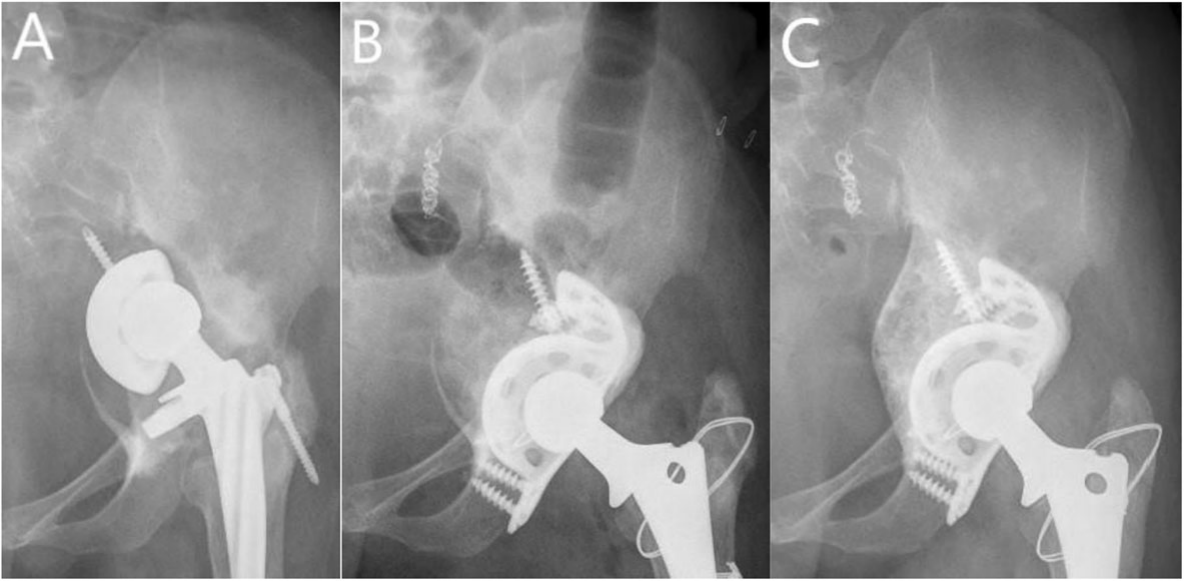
Radiographs of a 43-year-old woman with Paprosky IIIB acetabular bone defects and pelvic discontinuity. a Preoperative radiograph. b Immediate postoperative radiograph showed reconstruction cage and morselized allografts reconstructed the bone defect. c Radiograph at 96 months after revision suegery show that the cage remained stable and the allograft was completely incorporated with the host bone
